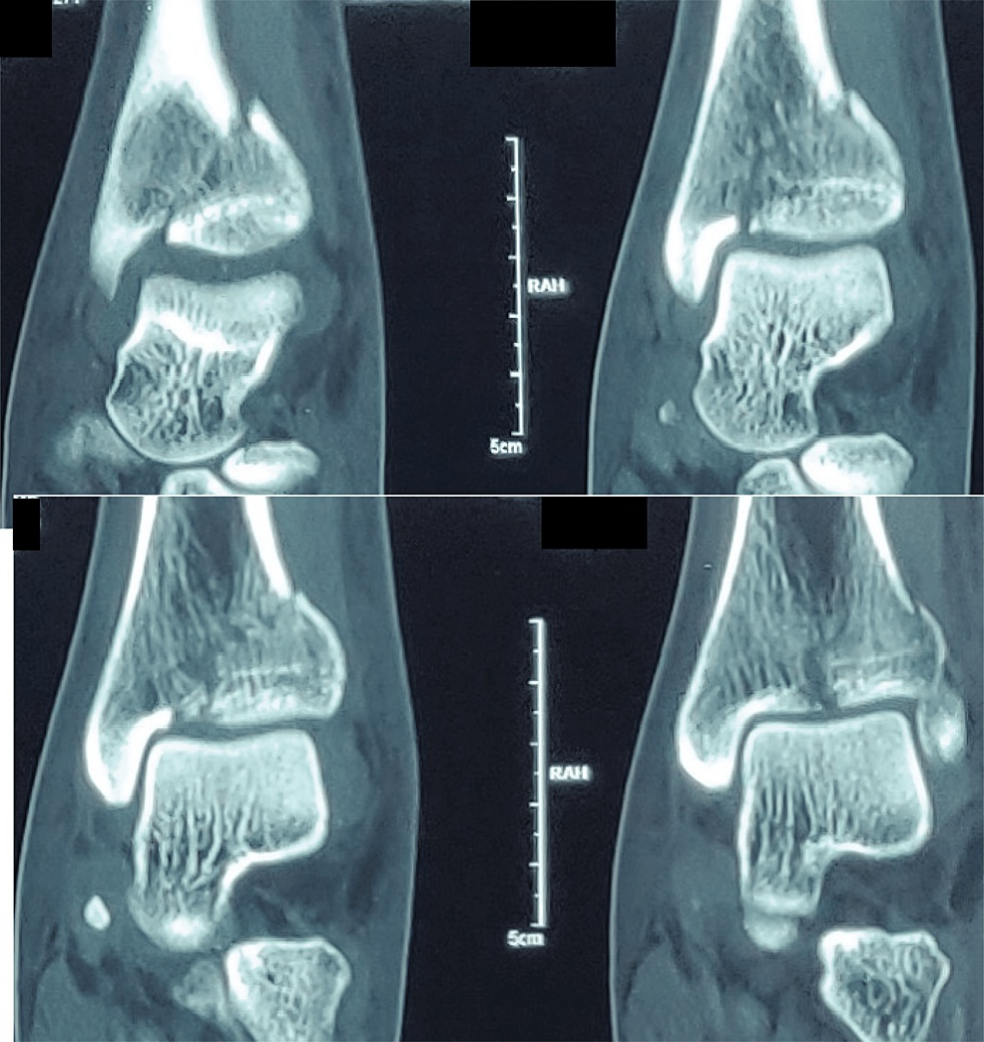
Coronal sections of CT scan of affected ankle
